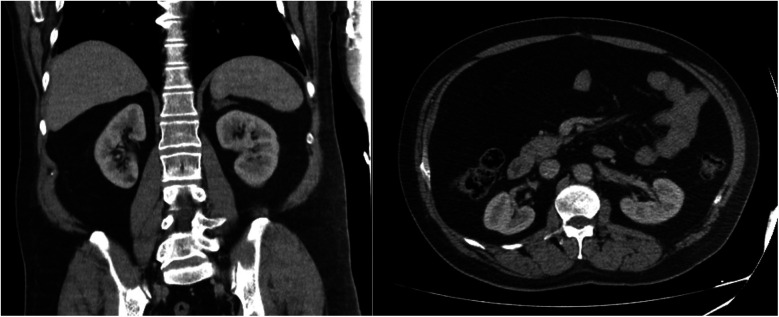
Computed tomography images at two years postoperatively showing successful resection of a giant adrenal myelolipoma.
